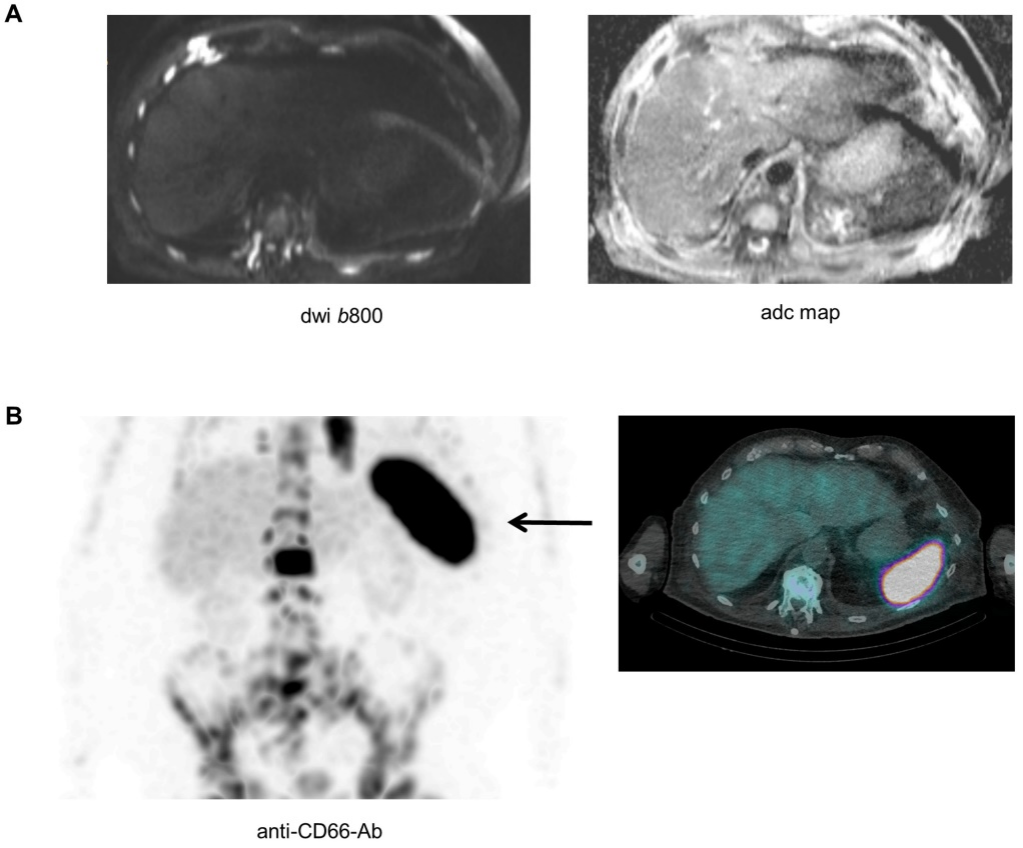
The asplenia phenomenon and extramedullary hematopoiesis. A patient with advanced MM and absence of spleen signal on DW-MRI also underwent Tc-99m labelled anti-CD66 antibody scintigraphy. In (A) the b800 DWI and ADC maps are shown. In (B) results of the Tc-99m labelled anti-CD66 antibody scintigraphy are depicted.
